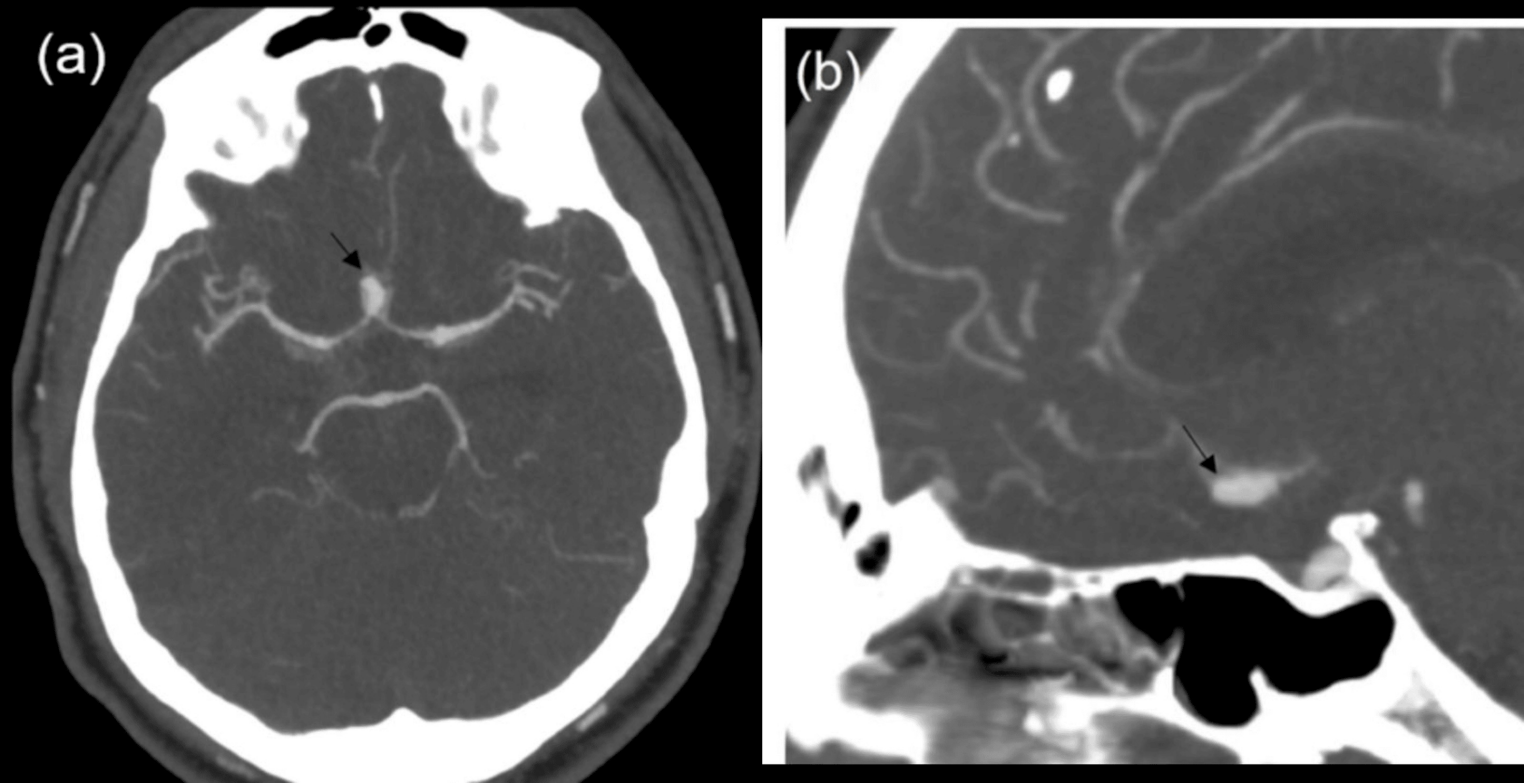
Computed tomography angiogram of brain Axial view (a) and sagittal view (b) show a saccular aneurysm directed anteriorly (black arrow) arising from the anterior communicating artery, which measures 0.9 x 0.6 cm.
